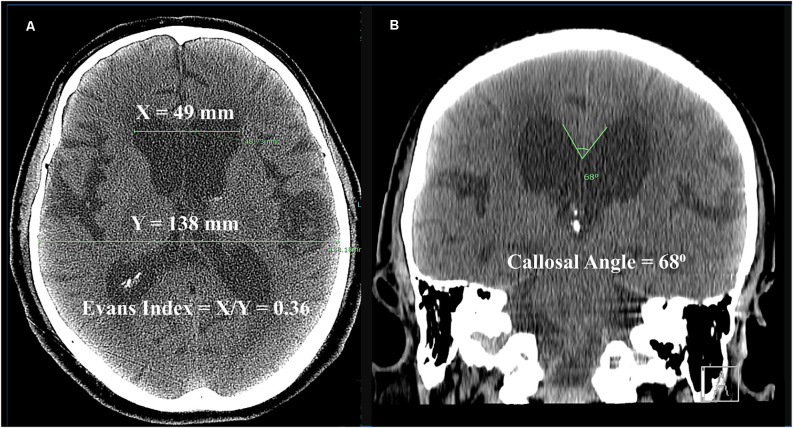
Computed tomography (CT) scan from a 70-year-old man with iNPH. (A) Axial image demonstrating Evans Index, which is the ratio of the maximum bifrontal horn width (X) to the maximum biparietal internal diameter of the skull (Y). An Evans Index >0.3 is present in iNPH. (B) Coronal image demonstrating callosal angle, here measured to 68 degrees. Normal callosal angles are greater than 90 degrees, while acute callosal angles occur in iNPH. Disproportionately enlarged subarachnoid space hydrocephalus (DESH) is apparent, as the Sylvian fissures are dilated out of proportion to sulci near the convexity.
